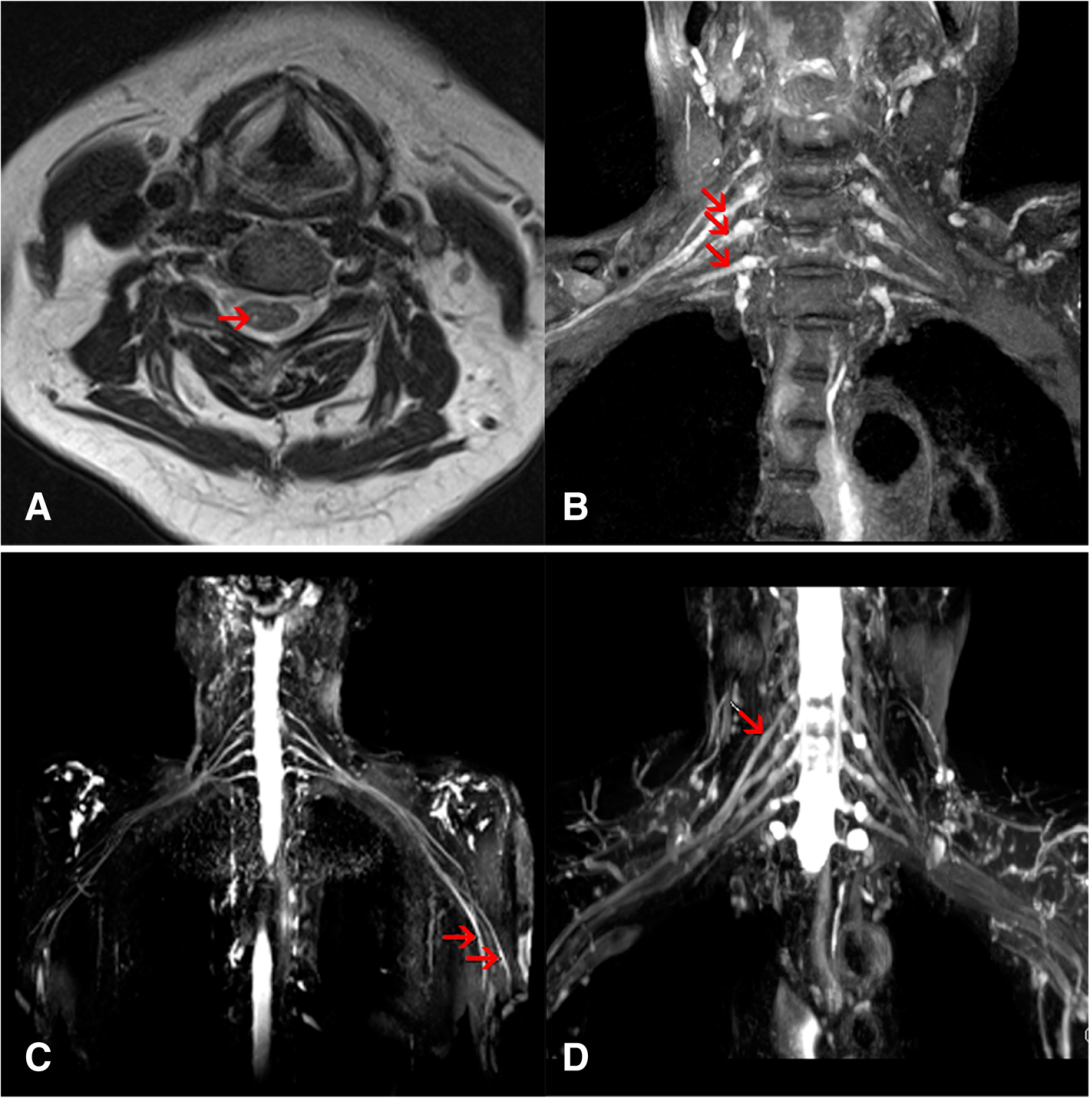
Imaging characteristics of patients with SZP. Axial T2-weighted image showed the unilateral hyperintensity in the dorsal horn of C5 spinal cord in patient 2 (a). Brachial plexus magnetic resonance imaging showed hyperintensity of C6–8 nerve roots in patient 5 (b), left median and radial nerves in patient 7 (c) and C5 nerve roots in patient 8 (d)
